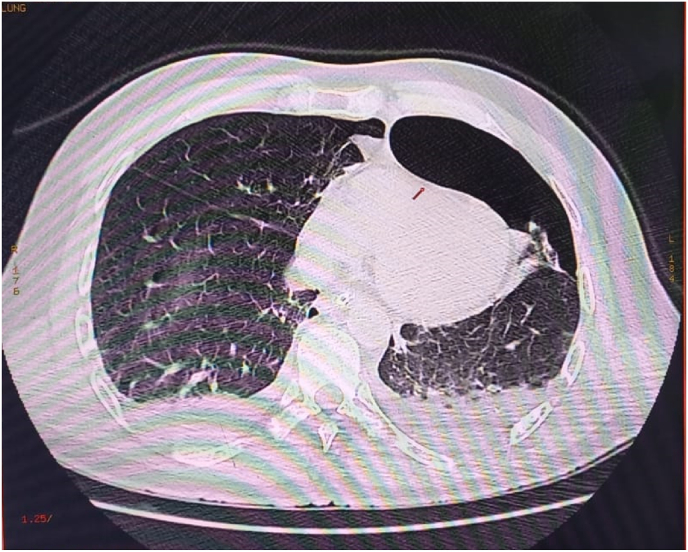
Axial sequence of a lung window chest computed tomography revealing a left pneumothorax with bilateral hemothorax.
